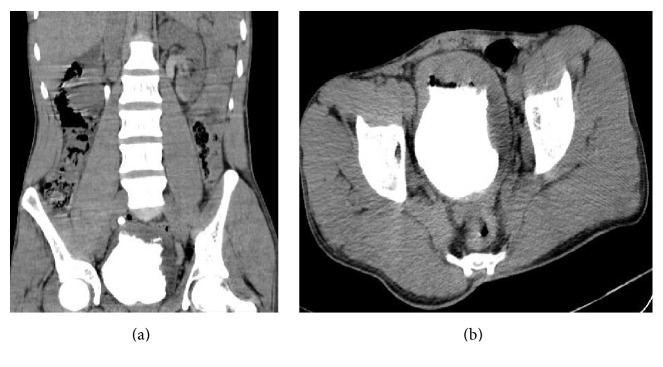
Abdominopelvic CT-scan of patient. (a) Axial view showing bilateral hydronephrosis and nonuniform decreased cortex; (b) horizontal view showing multiple filling defects in bladder.
